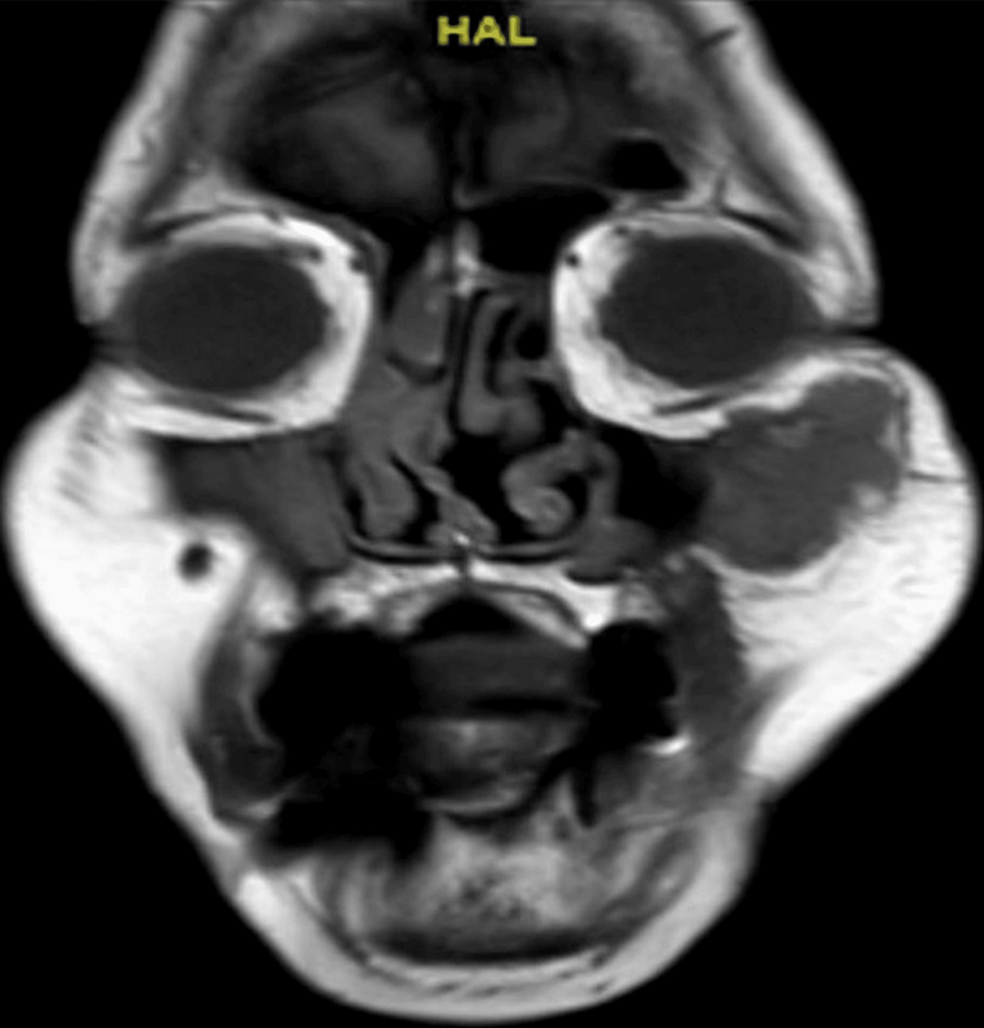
Head MRI shows well-defined lobulated soft tissue mass noted at the anterior left cheek subcutaneous area, with low to iso signal intensity
